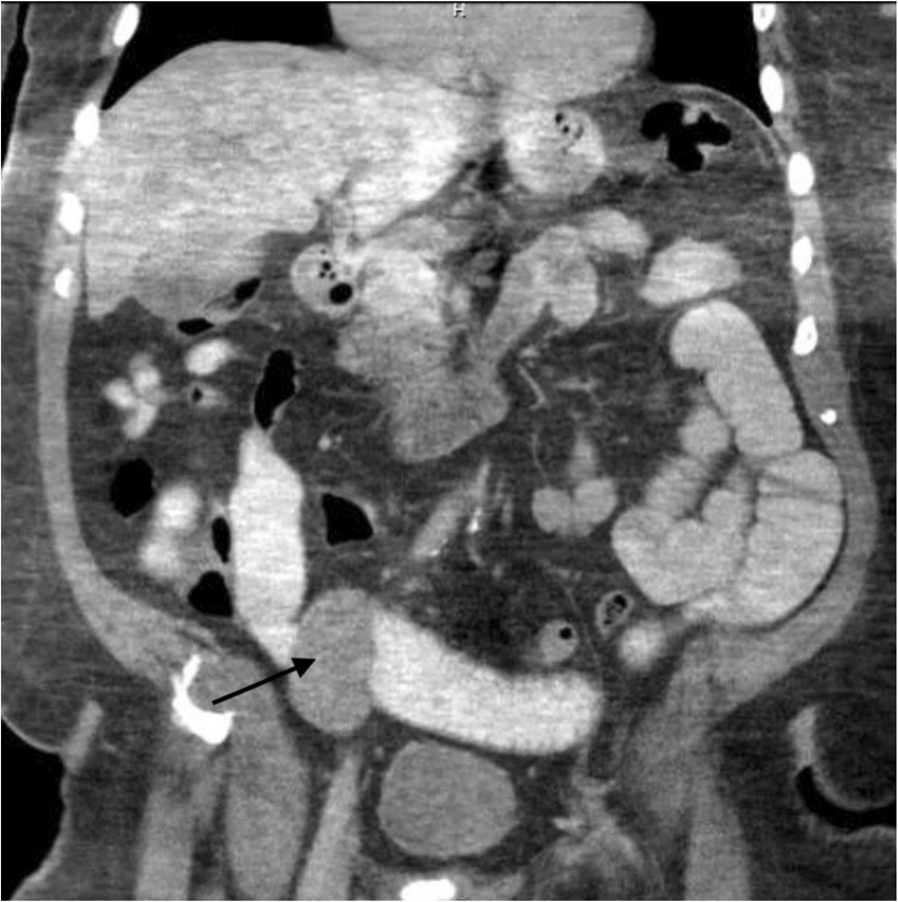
Coronal section of a computed tomography scan of the abdomen showing an area of soft tissue mass (*arrow*) in the small bowel in the right lower quadrant
